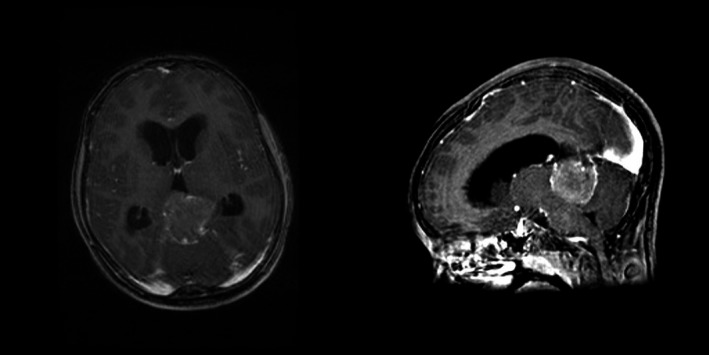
MRI of the brain: polynodular lesion in the epiphyseal region, partly calcified. Signs of hydrocephalus.
